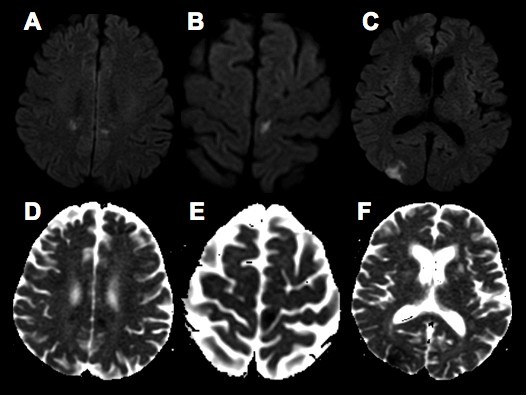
RVCS-related ischemic strokes. Diffusion-weighted MRI (A, B, C) and apparent diffusion coefficient maps (D, E, F) revealed lesions in the right occipital lobe and bilateral anterior cerebral artery territories consistent with ischemic strokes.
